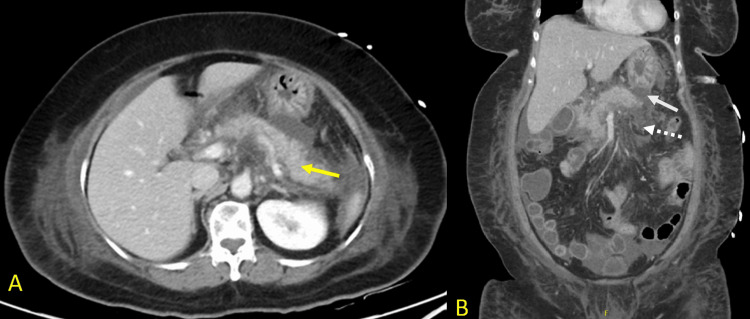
CT scan of the abdomen with contrast A: The axial section shows a diffusely enlarged pancreas (yellow arrow) that appears edematous with significant acute peripancreatic free fluid noted, measuring up to 30 mm in thickness. No evidence of parenchymal necrosis is seen. B: Coronal section, which shows the fluid extending superiorly into the lesser omentum (white arrow) and inferiorly along the inferior transverse mesocolon (dotted white arrow).
